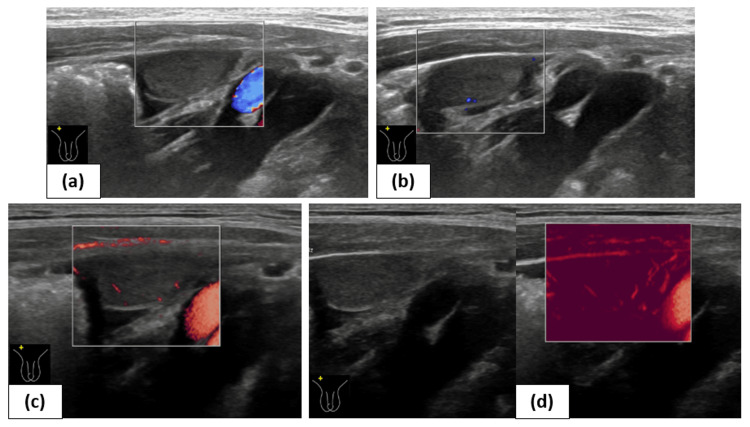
A two-year-old patient with swelling in right inguinal region. (a) CD shows grade 0 flow; (b) PD shows one spot color encoding signal, i.e., Grade 1 parenchymal flow; (c) cMVI shows a linear and two spot color encoding signals, i.e., Grade 2 flow; (d) mMVI shows multiple linear and spot color encoding signals, i.e., Grade 3 flow. CD: Color Doppler; PD: Power Doppler; cMVI: Color Microvascular Imaging; mMVI: Monochrome Microvascular Imaging
